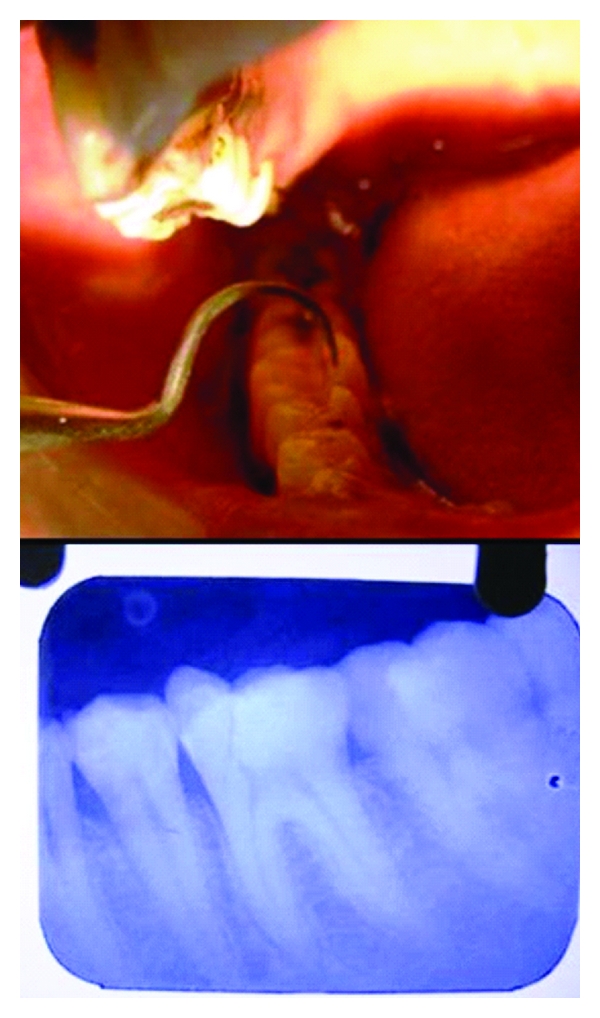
Vertical coronal fracture in mandibular molar, pre-operative radiograph.
